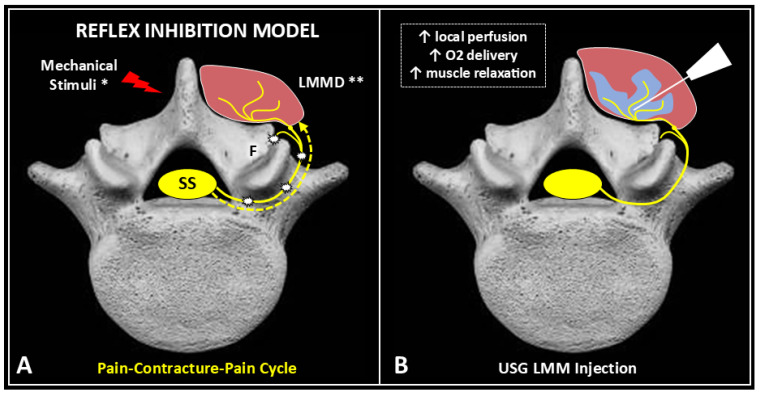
Mechanical stimuli (red flash) activate an inhibitory spinal reflex (A). Hyperactivation of intramuscular proprioceptors of the multifidus muscle (brown) generates continuous nociceptive afferent feedback (white flashes) via the dorsal ramus of the spinal nerve (yellow line), leading to efferent inhibitory signals (dotted curved yellow arrow) to the muscle fibers. Lumbar multifidus muscle dysfunction (LMMD) causes spinal sensitization (SS) as persistent contracture sustains afferent pain signals to the posterior horn of the spinal cord (yellow dot). Ultrasound-guided injection of the lumbar multifidus muscle (B) reactivates muscle fibers, counteracting spinal sensitization and interrupting the inhibitory reflex. F: facet; *: chronic overload of the vertebral segment; : chronic myofascial pain; light blue: injectate; white: needle.
